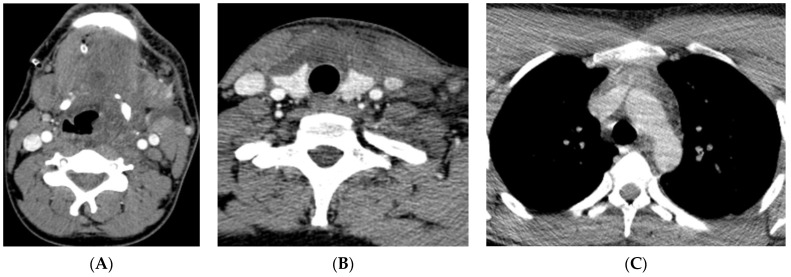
(Patient 1) Axial contrast-enhanced CT image; (A) abscess/hypodense lesions/in the left submandibular, parapharyngeal, pharyngeal mucosal and retropharyngeal spaces. (B) Mediastinitis in the visceral space. (C) Upper anterior mediastinum—mediastinitis.
